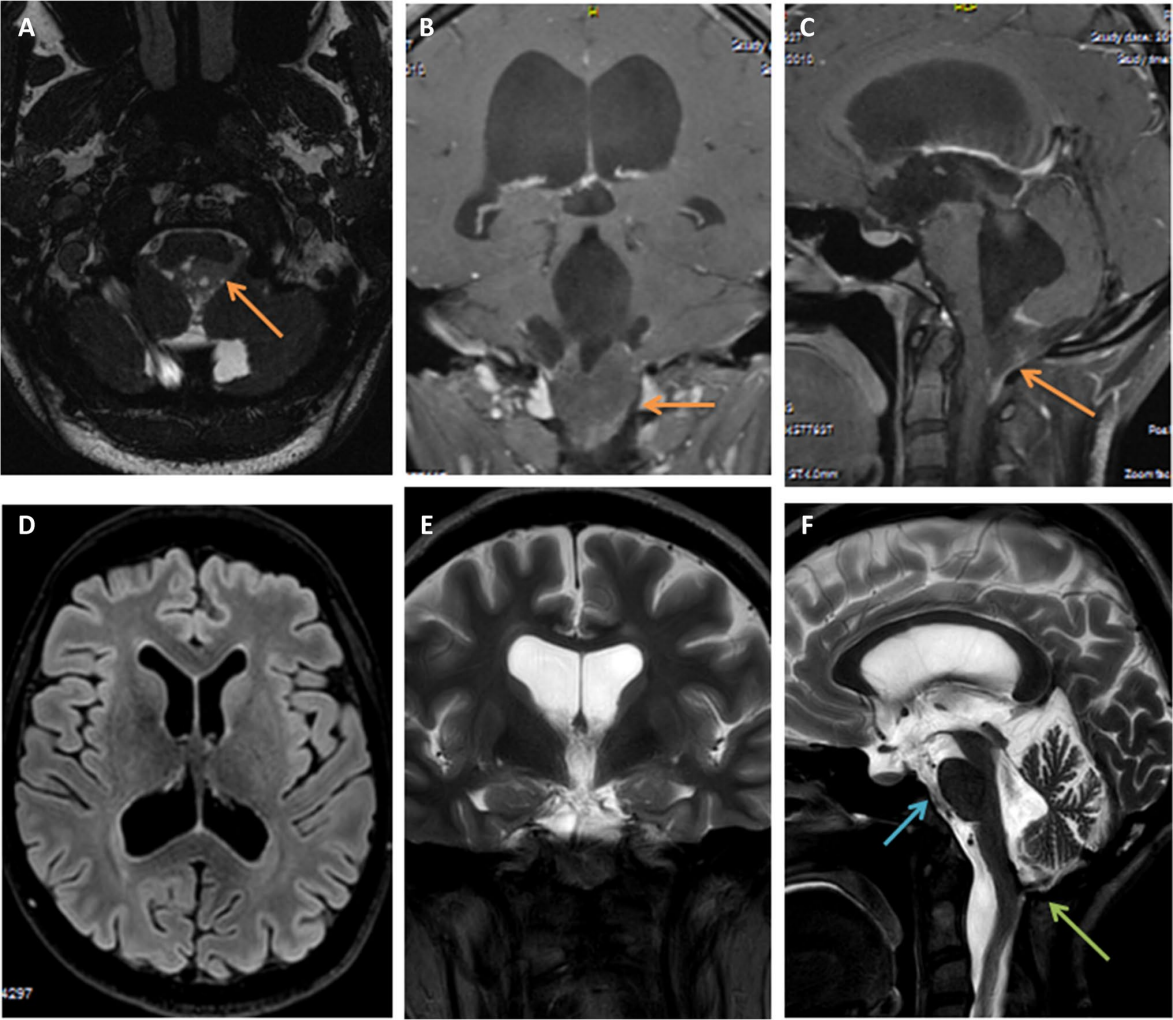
MRI scans performed in a 26-year-old woman with a 4th ventricle subependymoma. (A), (B) and (C) show axial fiesta-3D and contrast-enhanced T1-weighted coronal and sagittal images. Orange arrows show the tumour obliterating the foramina of the 4th ventricle with a subsequent obstructive four-ventricle HCP. (C), (D) and (F) demonstrate the FLAIR axial and T2-weighted coronal and sagittal MRI images after 10 years following ETV and tumour resection. Note that there is no tumour visible and no evidence of HCP (a marked decrease in Evans Index). The blue arrow in picture (F) shows the flow void phenomenon across the area of ventriculostomy, while the green arrow points to areas of possible postoperative adhesions (in black) at the level of the foramen of Magendie
